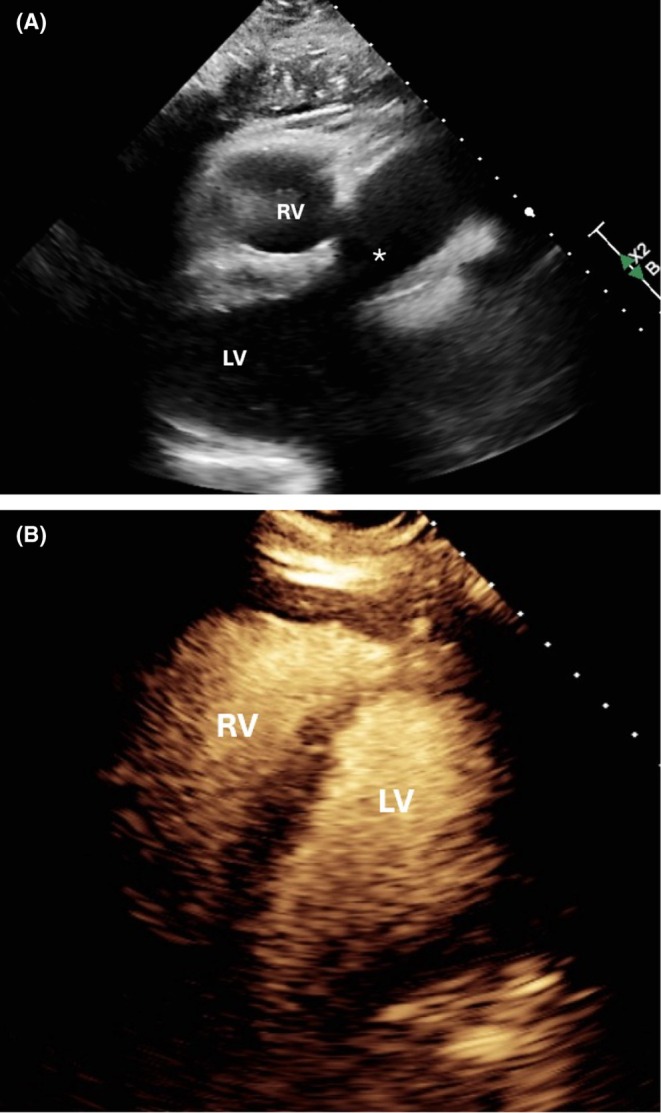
(A) Transthoracic echocardiogram (TTE) (parasternal long axis view) showing overriding aorta (marked by *). LV = left ventricle, RV = right ventricle. (B) Contrast images. LV, left ventricle; RV, right ventricle.
